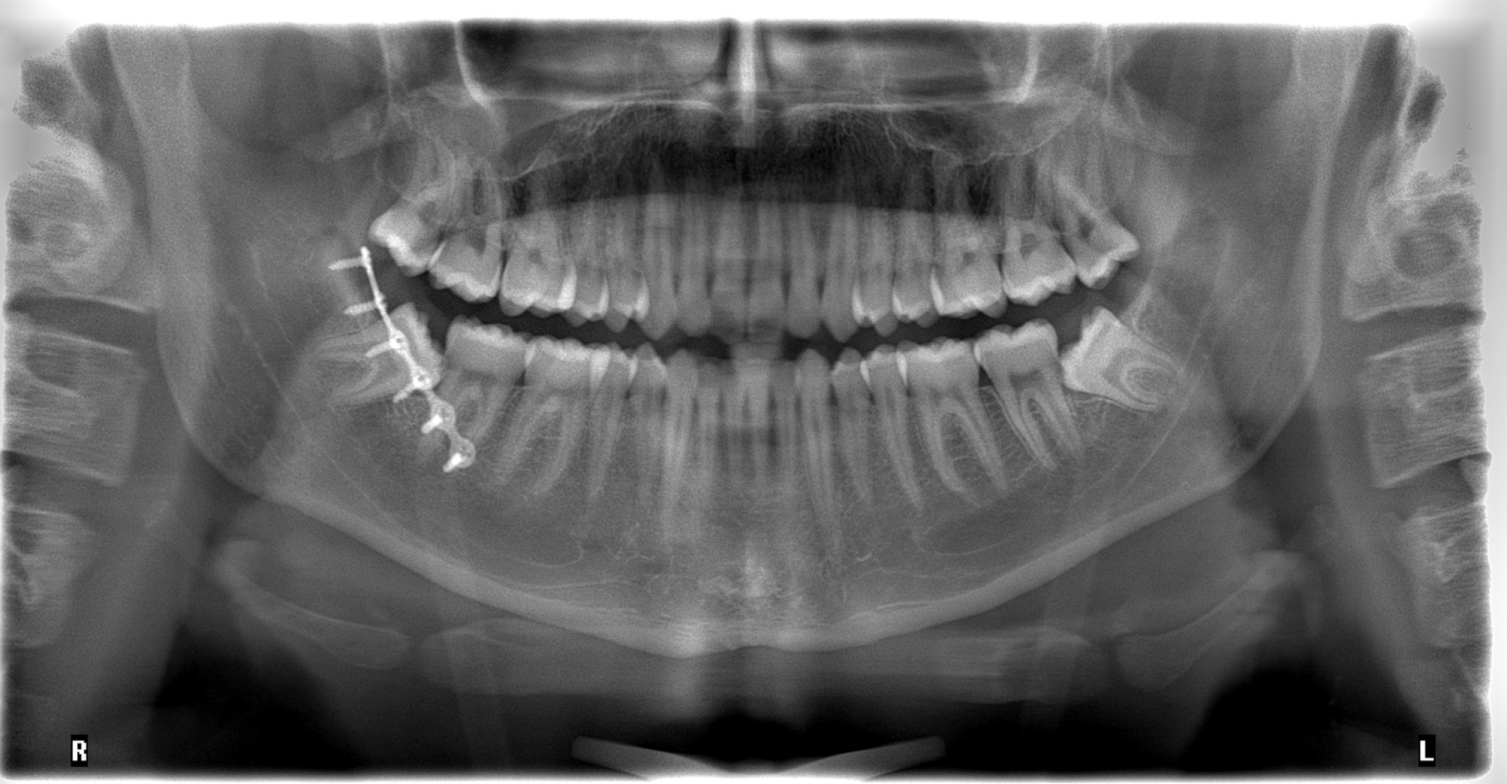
Postoperative orthopantomogram after repositioning of a right mandibular angle fracture and 1-plate fixation
